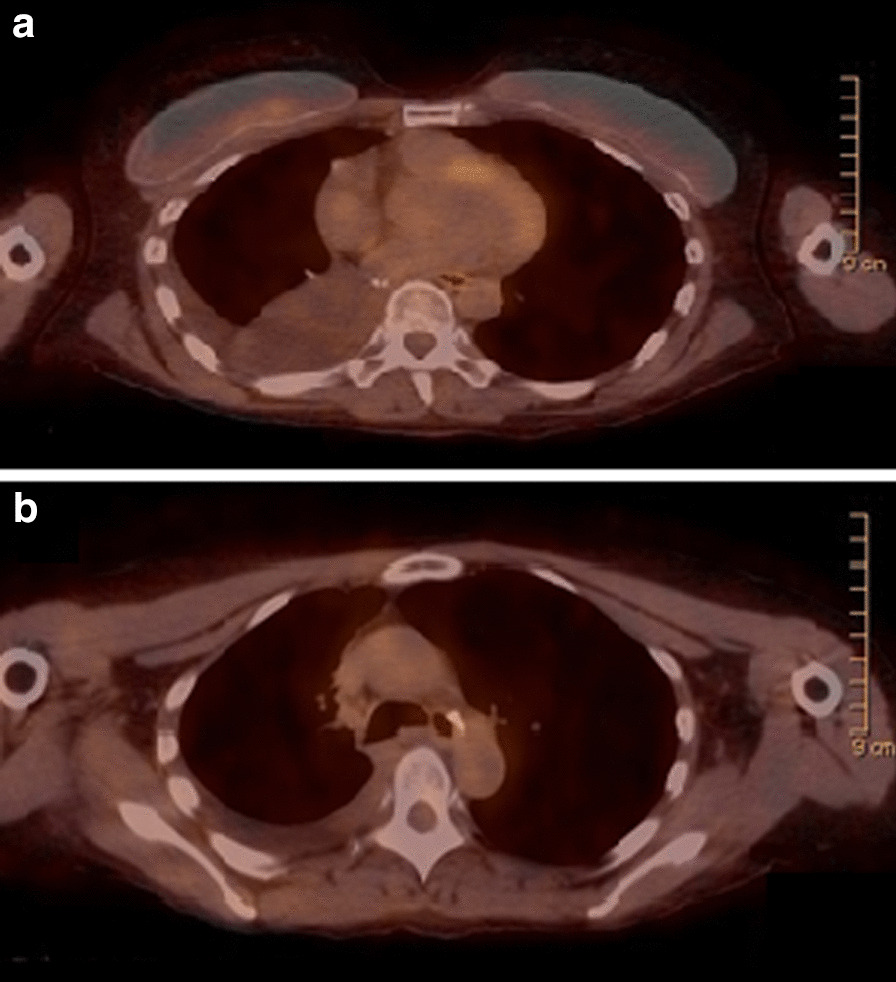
Disease status before and after neoadjuvant osimertinib therapy. a, b Positron emission tomography/computed tomography 10 months after diagnosis and 4 months after surgery. No evidence of disease
